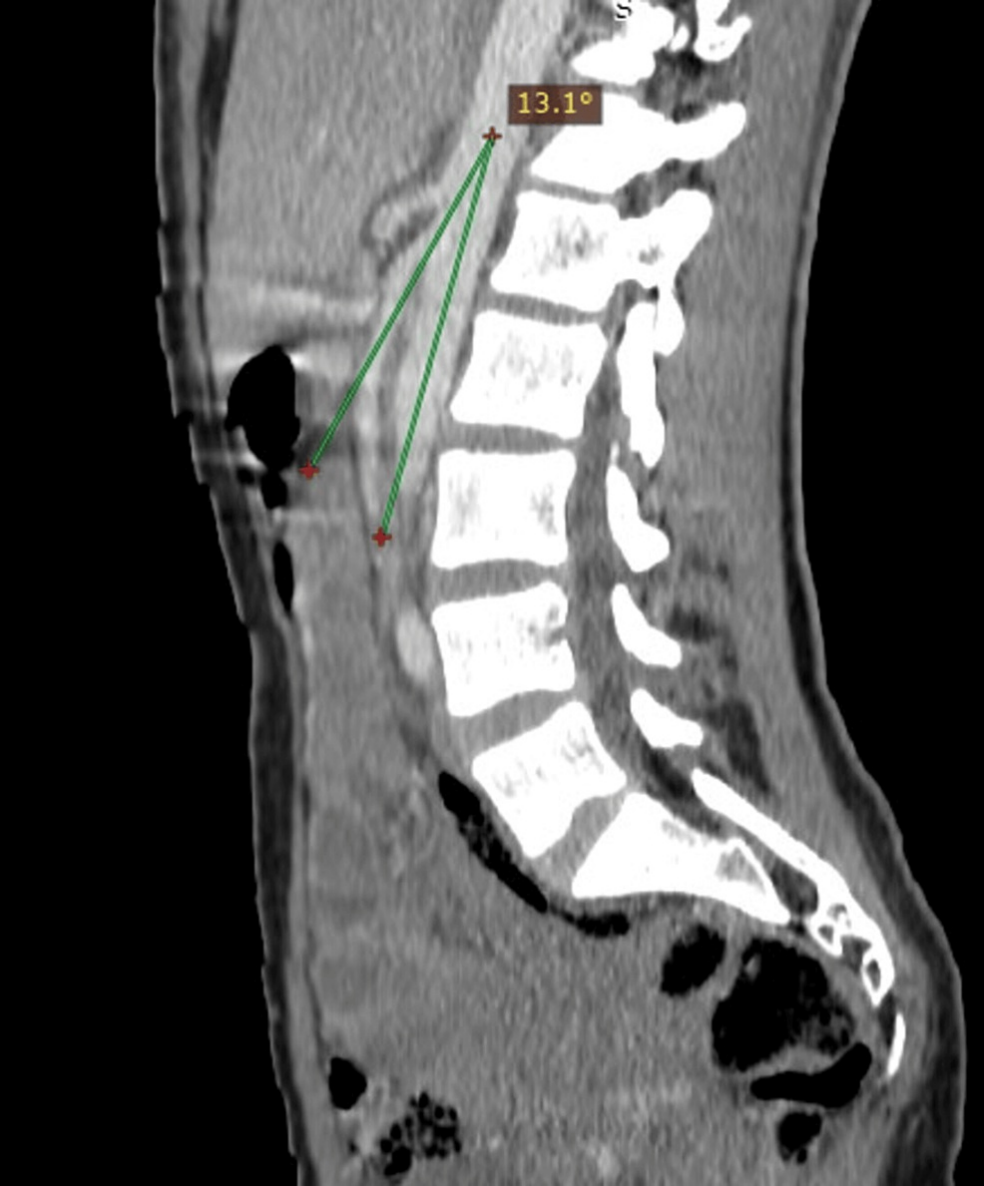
CT scan of the abdomen and pelvis (sagittal view) The image shows the angle between the abdominal aorta and the superior mesenteric artery which is measured at 13.1° (less than 41°), meeting the diagnostic criteria for Nutcracker Syndrome (NCS).
